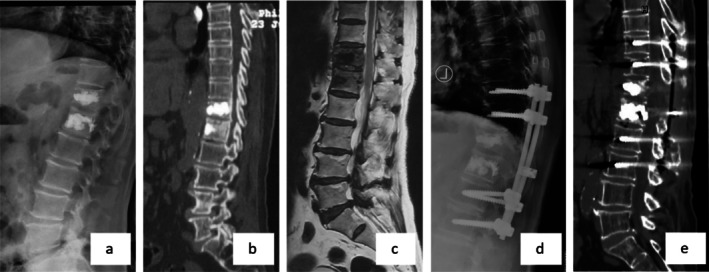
A 66‐year‐old female patient diagnosed with T12–L1 vertebral tuberculosis after T12–L1 vertebral augmentation. (a–c) Preoperative X‐ray, CT and MRI showed that there was bone destruction in the T12–L1 vertebrae and intervertebral space; the bone cement filled the vertebral body well, with good stability, and that no bone cement protruded into the spinal canal to compress the spinal cord. After 2 weeks of standard antituberculosis drug treatment, posterior thoracic canal decompression, bone graft fusion and internal fixation were performed; (d and e) Reexamination X‐ray and CT 18 months after the operation showed that the internal fixation was in good position without obvious loosening, no further bone destruction was found in the T12–L1 vertebrae compared with the preoperative period, and the lesion healed well.
